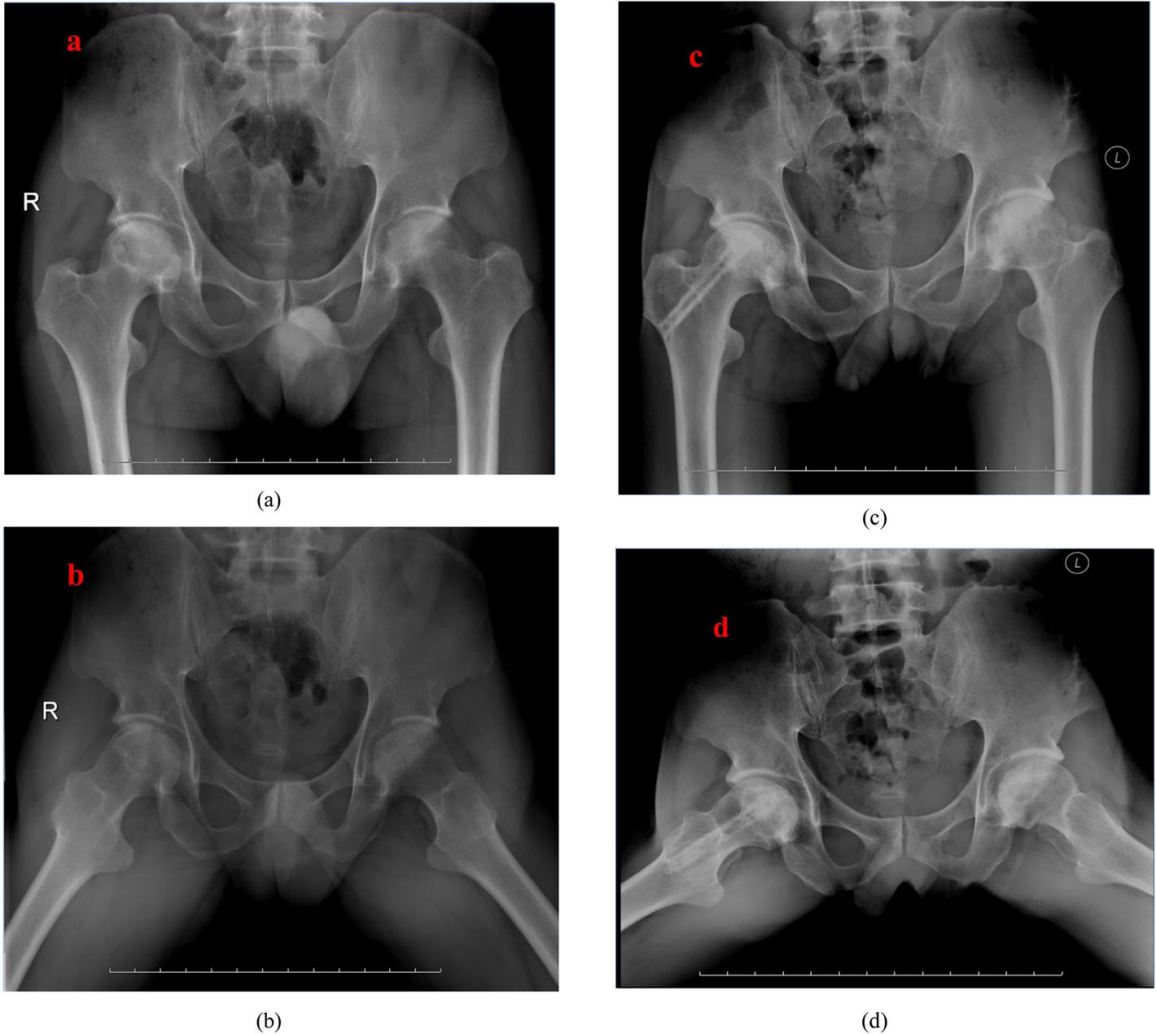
a, b 41-year-old male patient complained bilateral hip pain for 2 years. c, d The patient accepted one-stage fibular impaction allografting in the right hip and vascularized greater trochanter flap with the combination of cortico-cancellous iliac bone autografting. X-rays showed completed new bone formation and normal femoral head morphology without the presence of osteoarthritis at postoperative 8-year follow-up
